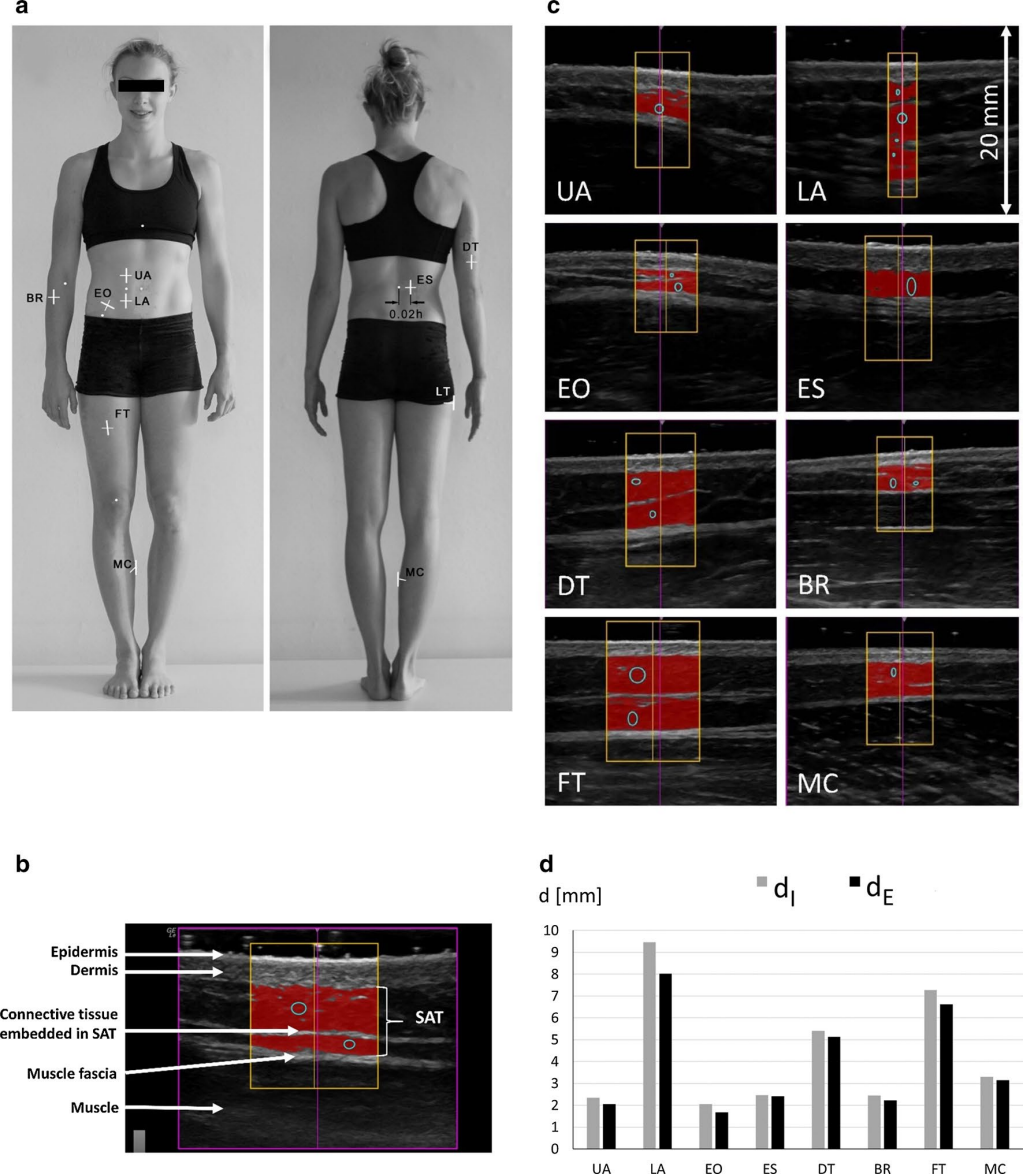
Standardised ultrasound (US) measurement of subcutaneous adipose tissue (SAT). a US sites. *UA* upper abdomen, *LA* lower abdomen, *EO* external oblique, *ES* erector spinae, *DT* distal triceps, *BR* brachioradialis, *FT* front thigh, and *MC* medial calf. Instead of the now standard lateral thigh (LT) site, the EO site was in use when this study was performed. For a detailed description of site marking, see [6, 7]. b B-mode US image of SAT. All eight sites show the same structure: the black band on top represents the thick gel layer (to avoid tissue compression), followed by the structures described in the figure. The amount of embedded fibrous structures (connective tissue) varies between individuals and from site to site; this holds also true for the skin thickness. c Evaluated US image series. Red areas represent the SAT detected by the semi-automatic contour detection algorithm [3, 6]; the ellipses indicate the regions where the algorithm started out for SAT contour detection. In this image series, the evaluation software determined between 48 (LA) to 155 (FT) thickness values within the rectangular ROI. d Survey of results: SAT patterning. SAT thicknesses with the embedded fibrous structures included (d_I_), and excluded (d_E_). The sum of the eight d values is termed D. In this participant, D_I_ was 34.8 mm, D_E_ was 31.3 mm, and about 10% of the mean depth comprised fibrous structures, while 90% was lipid, water, and adipose cell structures. BMI of this athlete was 22.0 kg m^−2^, and MI was 21.7 kg m^−2^
